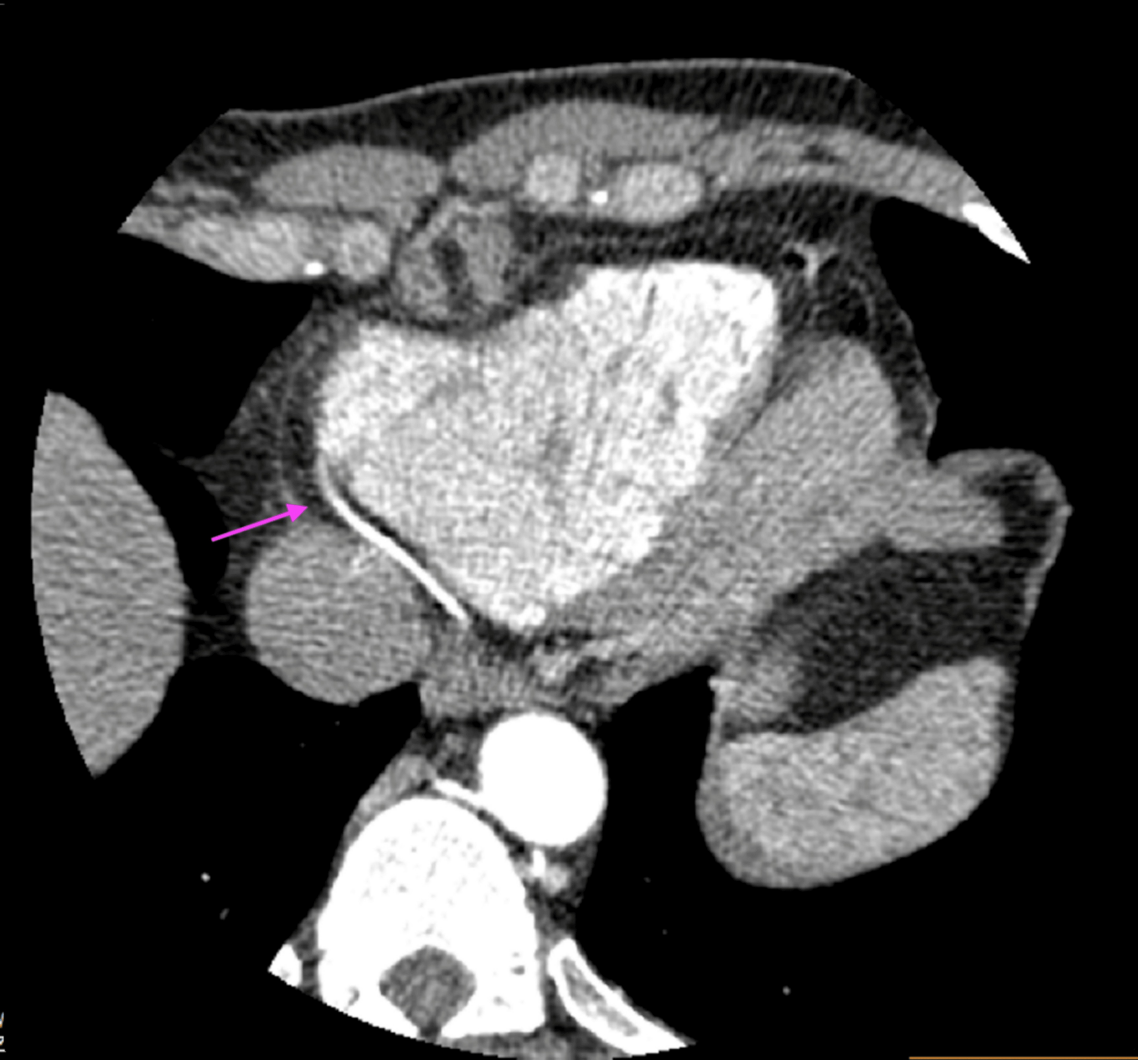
CT angiogram showing the anomalous origin of the RCA CT, computed tomography; RCA, right coronary artery
